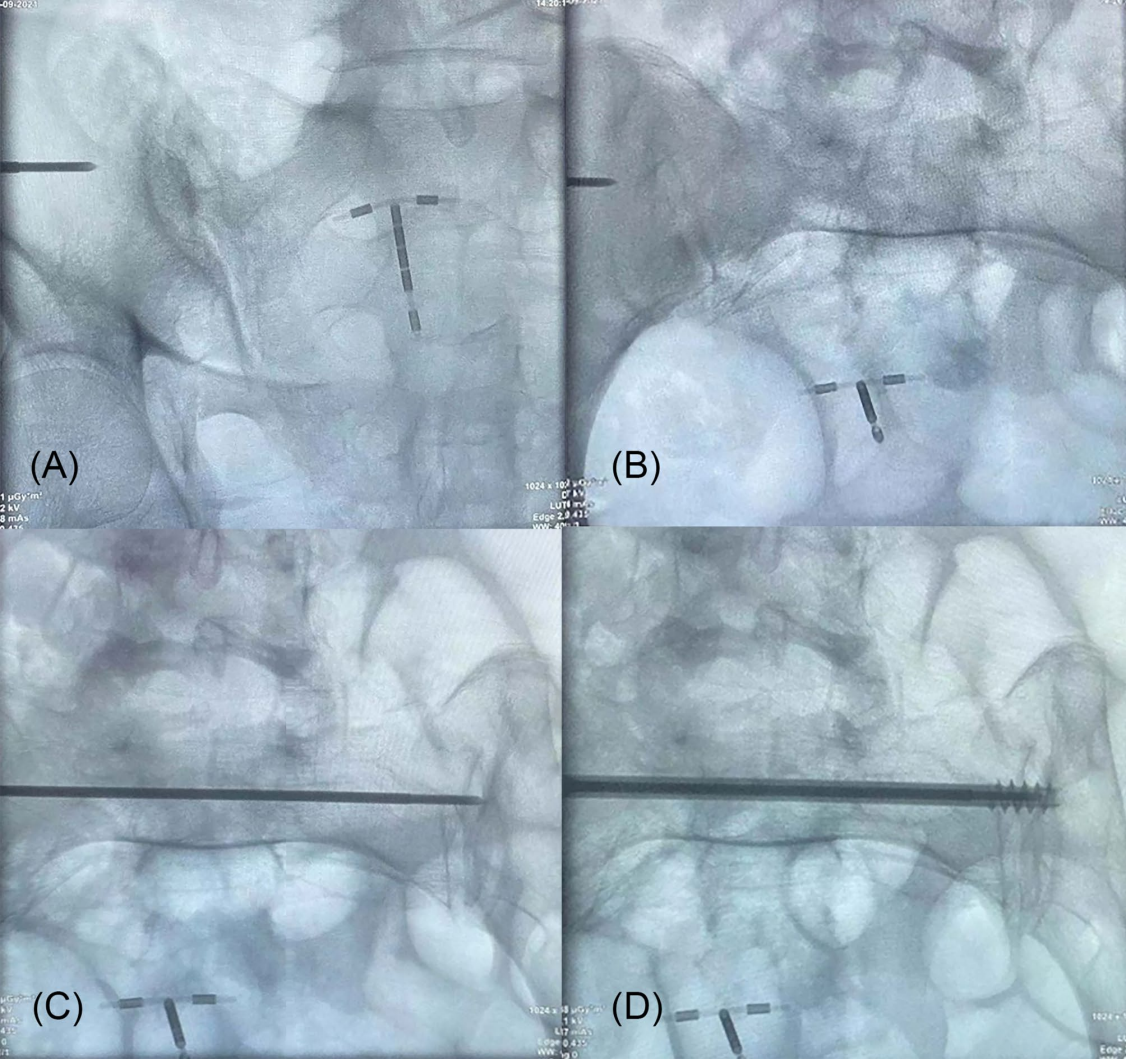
Radiographic imaging of the processes in sacroiliac screw fixation. (A) Pelvic outlet view. (B) Pelvic inlet view. (C) Placement of guided pin. (D) Placement of the sacroiliac screw in S1 across the bilateral sacroiliac joints.
